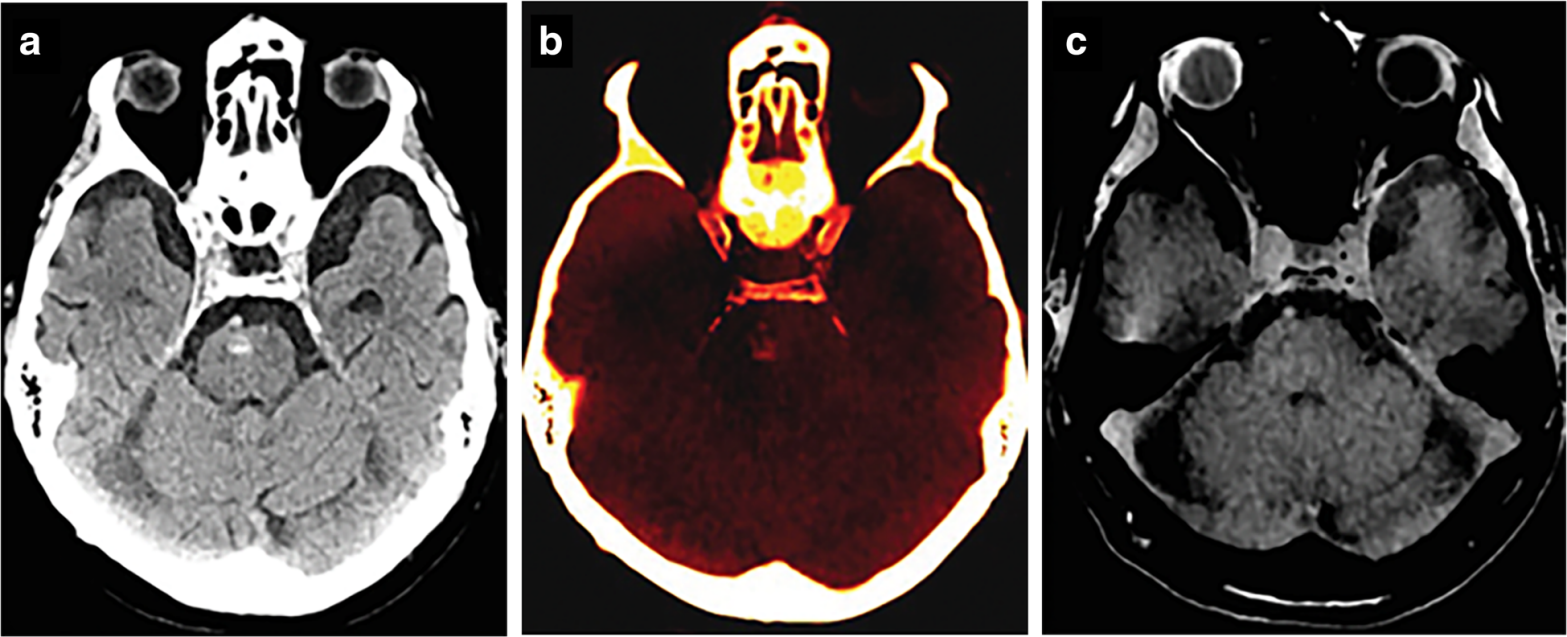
Head dual-energy computed tomography scan shows a focal hyperattenuation in the pons classified as indeterminate at standard grayscale series (a). This 80-year-old patient with a history of hypertension presented to the emergency department with altered mental status. On the calcium-overlay image (b), the hyperattenuation focus remains visible, while it is not apparent on the virtual non-calcium image (c), suggestive for parenchymal calcification. The finding was confirmed by magnetic resonance imaging. From Hu et al. [17]
